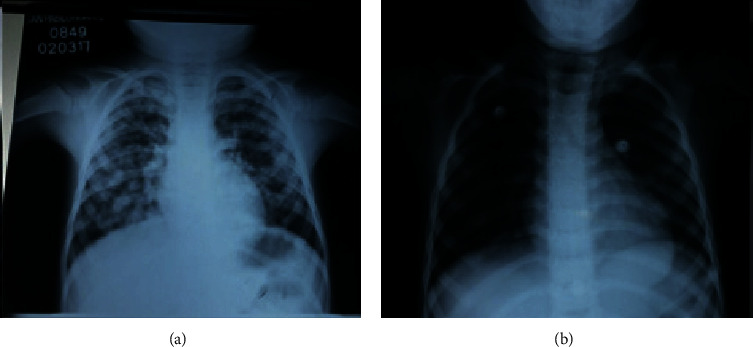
X-ray upon admission to the emergency unit (a) and prior to discharge of the same case (b).
